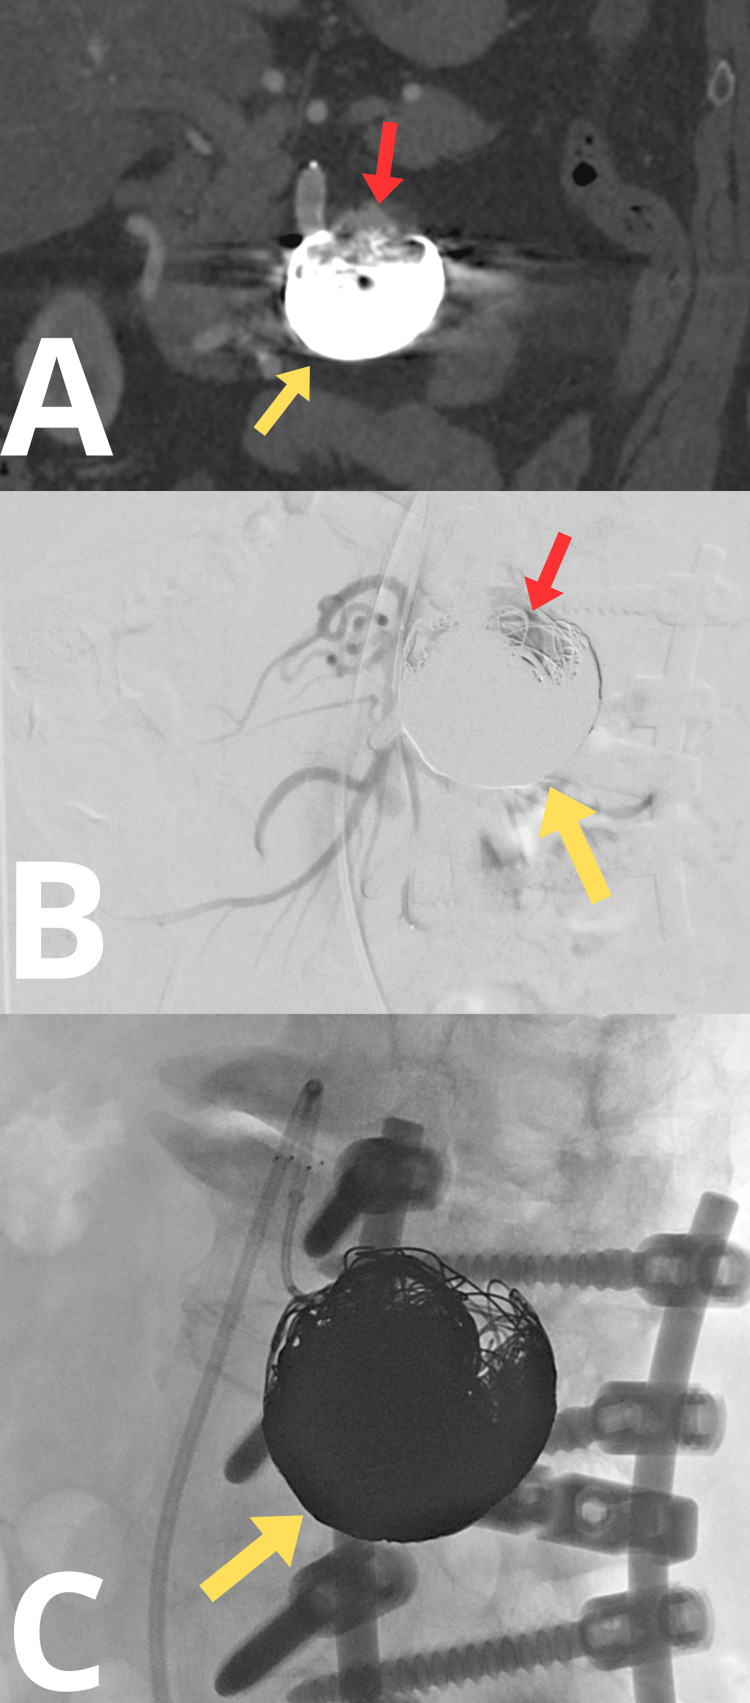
Angio-CT scans. (A) Visible contrast in the aneurysmal sac during the follow-up. (B) Intraoperative angio-CT. (C) Final embolization result. The yellow arrow indicates embolization coils. The red arrow indicates the trace filling portion of the aneurysmal sac.
